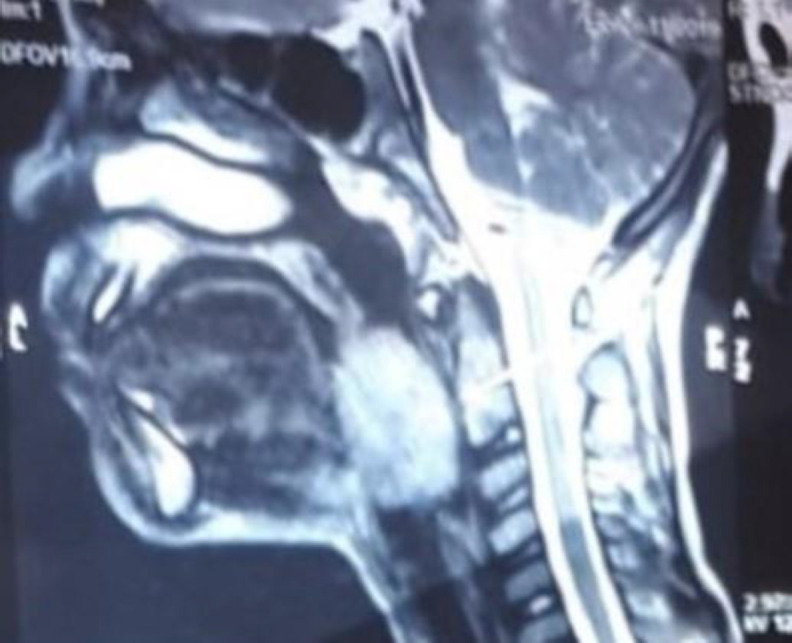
CT neck showing hyperdense lesion involving epiglottis extending into vallecula obstructing the airway
